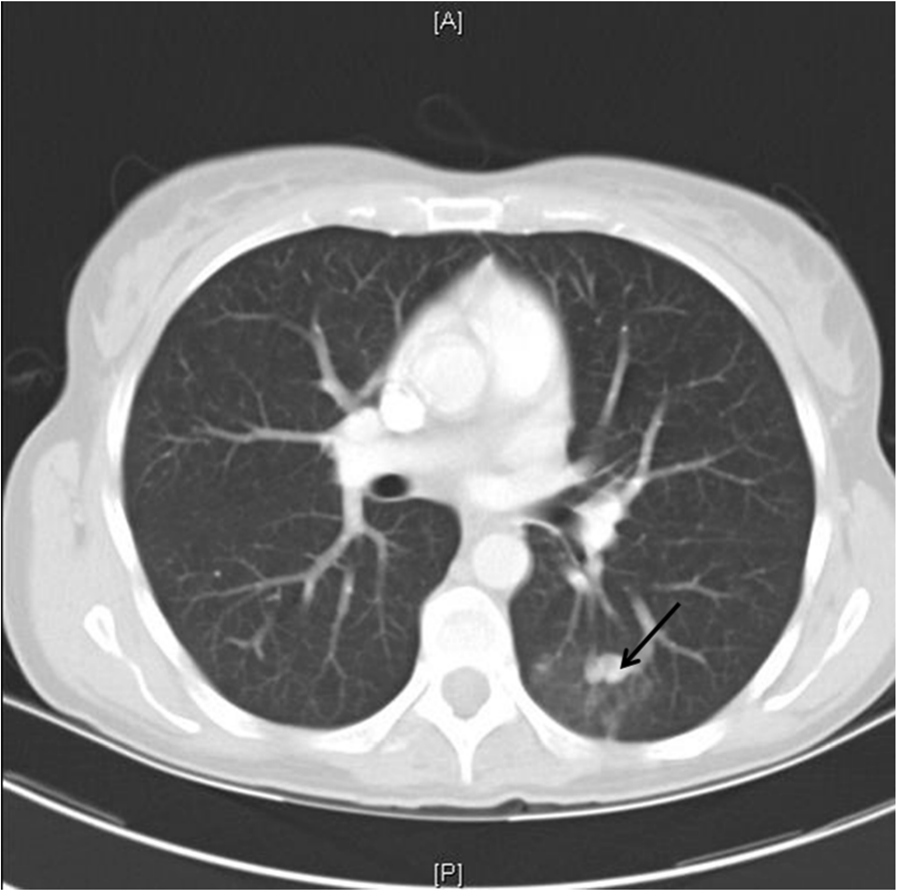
Axial computed tomography image at level of aortic root demonstrates small left lower lobe pulmonary arteriovenous malformation (arrow) with surrounding ground glass opacity consistent with recent haemorrhage.
